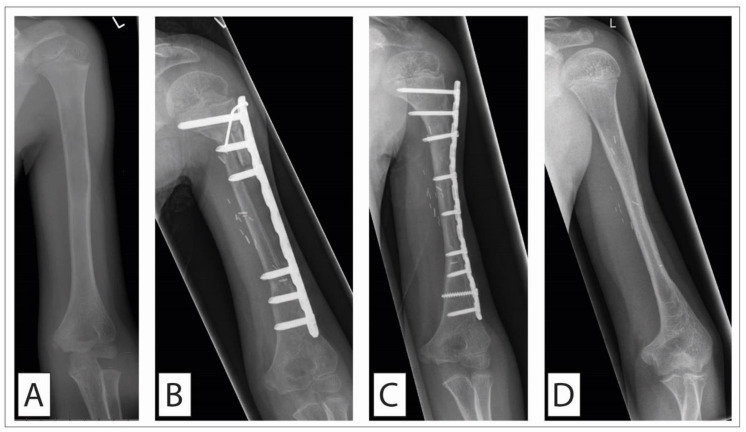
Ewing sarcoma of the humerus treated with a vascularized fibula graft. A 6-year old boy presented in the ward with persisting pain for 2 months in the left upper arm accompanied by intermittent fever. The plain a-p radiograph shows the central osteolysis within the diaphysis (A). Open biopsy was performed, showing Ewing sarcoma. After neoadjuvant chemotherapy, we performed complete en bloc resection (R0). The defect was reconstructed using a vascularized fibula graft by our colleagues from the department of plastic surgery (B). Temporary stabilization was performed with an LC-DCP plating. Seven months postoperatively, the fibula appeared radiologically fully osseointegrated distally, but a non-union with fracture of the plate was present proximally between the second and third screw. The LC-DCP was removed, and a titan elastic nail inserted. Postoperative care was a total cast of the left arm. Three months postoperatively, autologous bone from the iliac crest was placed at the non-union site followed by again plating of the humerus (C). (D) One year thereafter, the osteosynthesis material could be completely removed. The patient could freely use his arm, with, in the plain a-p radiograph, only a slimmer silhouette of the diaphysis still reminding of the performed procedure. Even when using a vascularized bone, as in the present case, bone healing can be challenging due to the immediate continuation of chemotherapy after wound healing.
